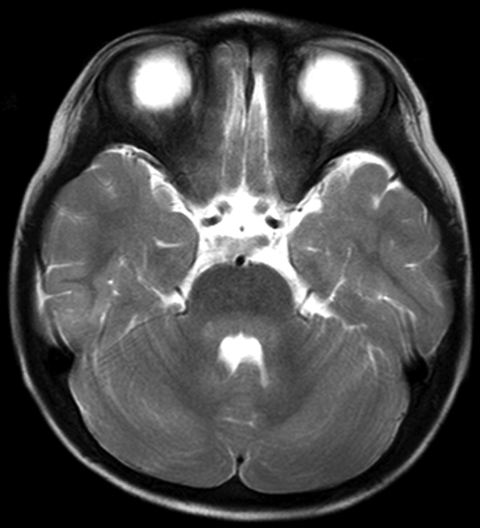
Axial T2-weighted slice of brain by magnetic resonance imaging, showing hyperintensity lesions in the pons and cerebellum around the fourth ventricle.
